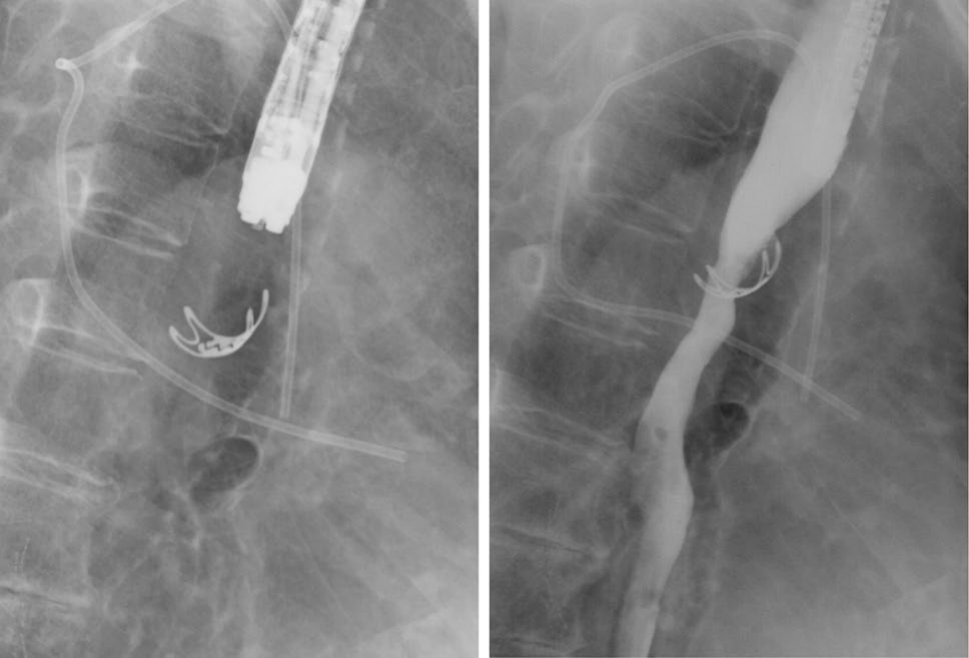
Non-contrast radiography showing the OTSC system in use (*left*) and contrast radiography showing no leakage (*right*)
